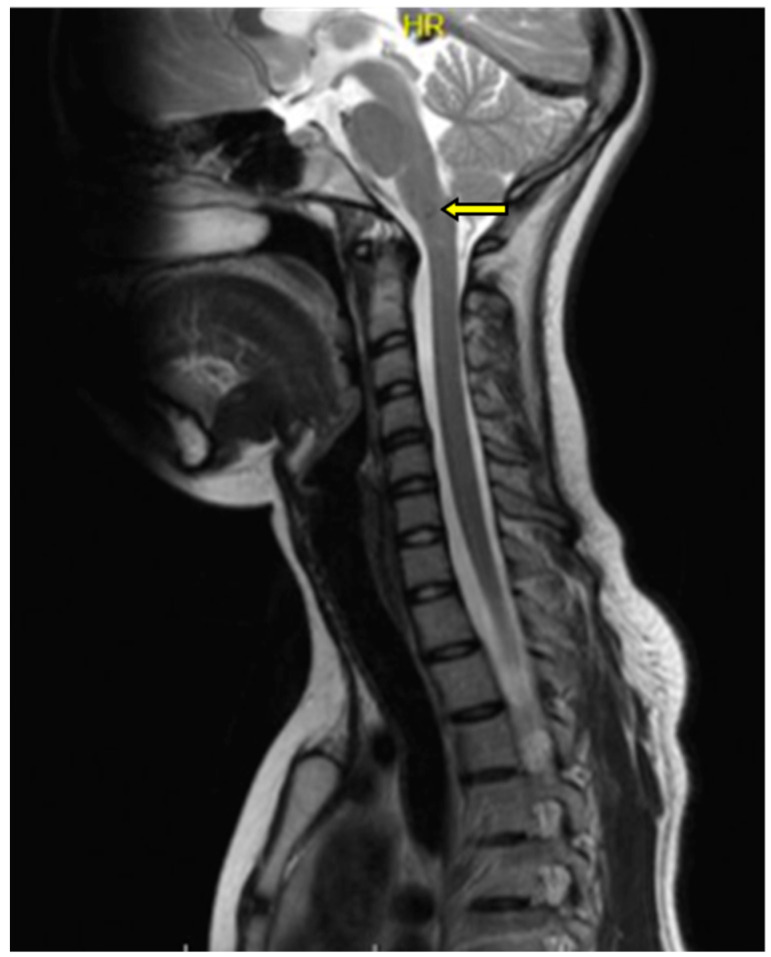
Magnetic resonance imaging (MRI) examination of the cervical spine in the T2 sequence. In the cervical spinal cord, no pathologies were seen. Within the medulla oblongata, a 2 mm lesion with a heterogeneous signal was visualized; pathologic change was marked with an arrow.
